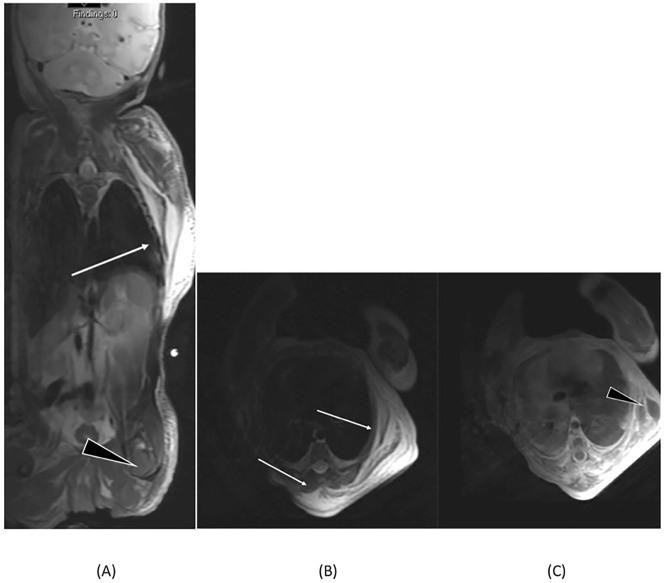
MRI exam. Coronal T2-weighted fat-saturated MRI reveals T2 hyperintense fluid collections (arrow) extending to the left leg’s subcutaneous fat (arrowhead) (A). T2-weighted fat-saturated MRI (B) and T1-weighted post-contrast fat-saturated scan (C) indicate fluid collections (arrow) in the left posterior chest wall without contrast enhancement (arrowhead).
